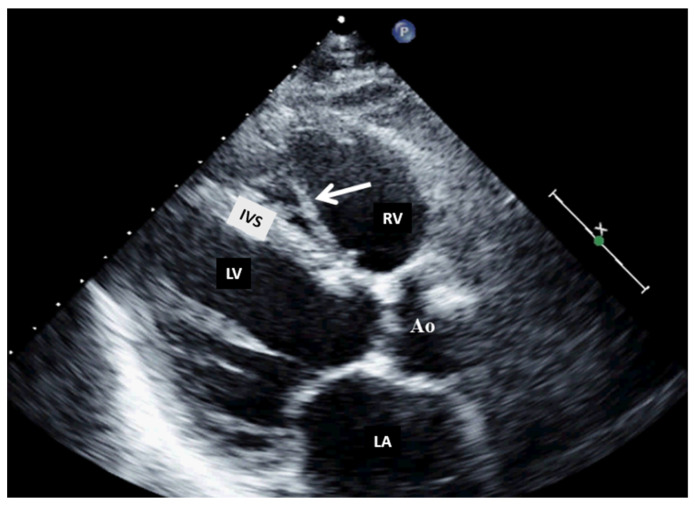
Two-dimensional transthoracic echocardiography (modified parasternal view, long axis) showing a moderator band in the right ventricle. Ao, aorta; IVS, interventricular septum; LA, left atrium; LV, left ventricle; RV, right ventricle.
